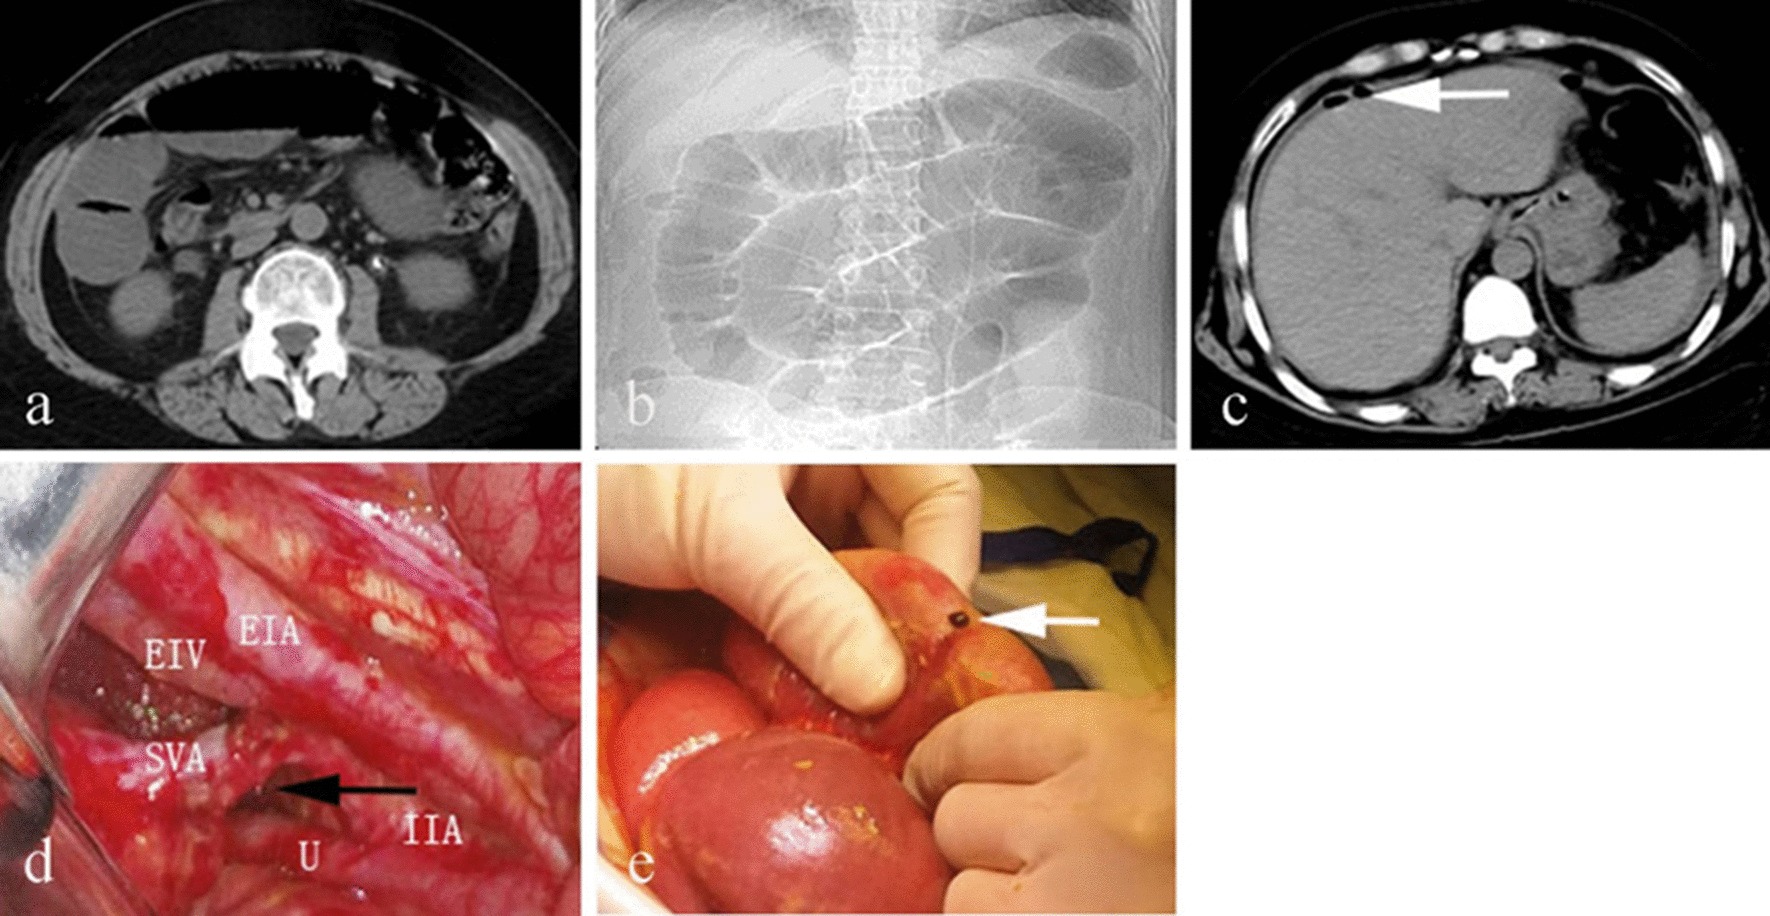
a CT scan revealed small intestine gas–liquid plane. b Small bowel obstruction. c White arrow for free gas in the abdominal cavity. d
